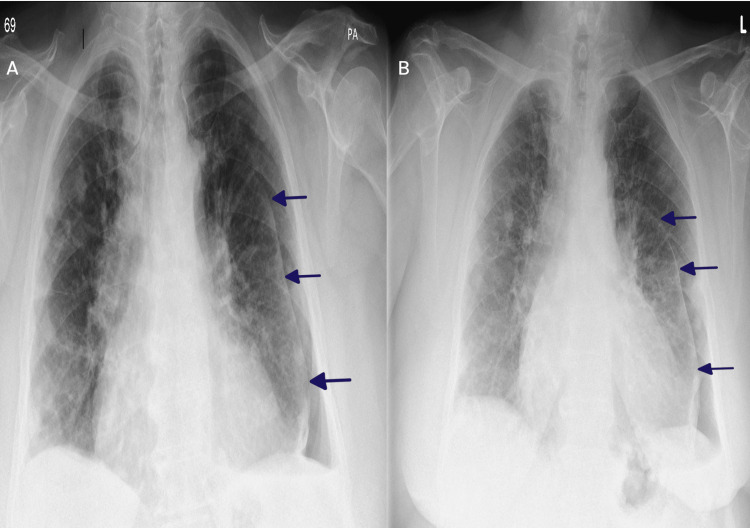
Serial chest X-rays (posteroanterior view) demonstrating left-sided hydropneumothorax. A) Initial image from the respiratory clinic showing a left-sided hydropneumothorax. B) Follow-up image from the pleural clinic showing a marginal increase in the pneumothorax size Blue arrow: Lung margin
